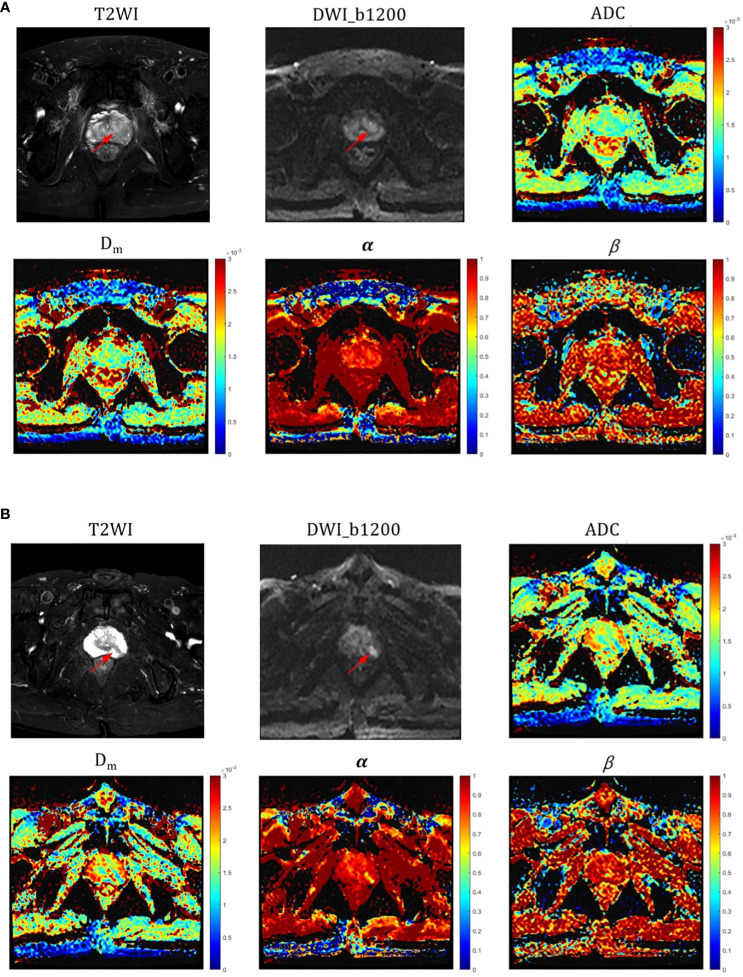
(A) Representative images (T_2_WI, DWI with b-value=1200, ADC, Dm, α and β) for a male patient with CP (red arrow pointing to); (B) Representative images (T_2_WI, DWI with b-value=1200, ADC, Dm, α and β) for a male patient with PCa (red arrow pointing to). ADC, α, and Dm values were all significantly different in CP and pCa, especially in α.
